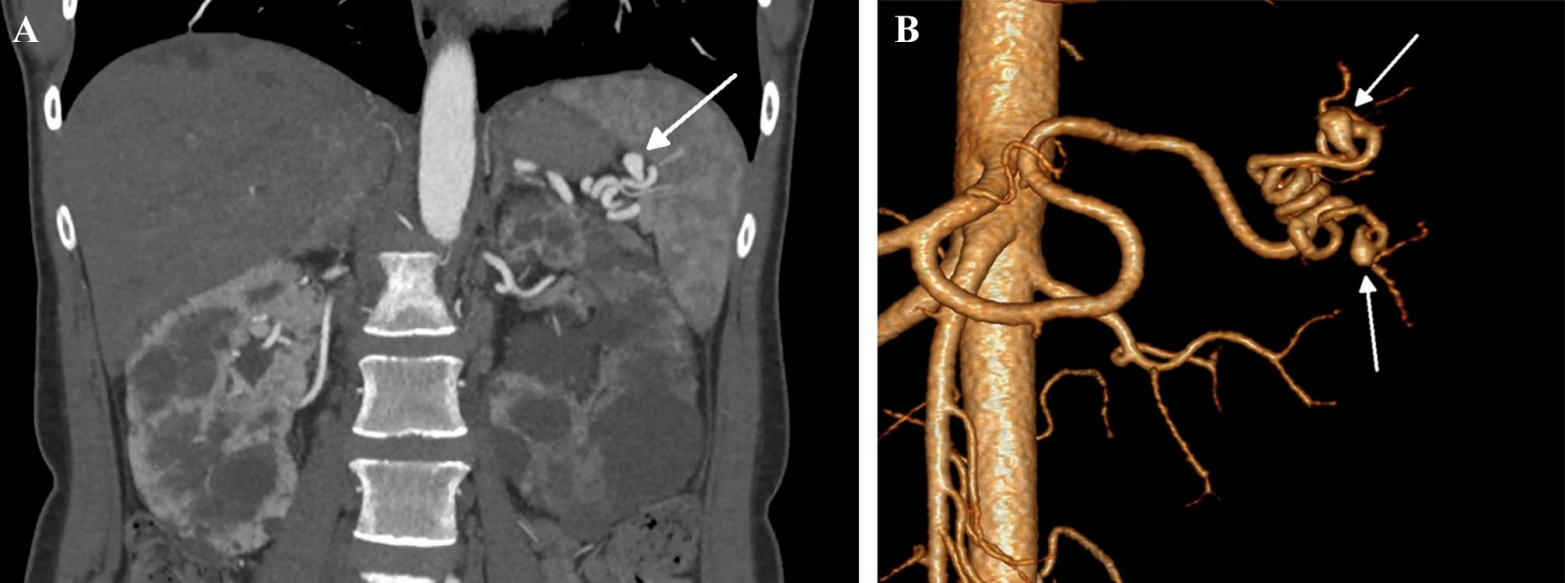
A Contrast-enhanced computed tomography of the abdominal cavity, coronal plane. Aneurysm of the splenic artery (arrow). Polycystic kidneys. B Contrast-enhanced computed tomography (3D reconstruction). Two aneurysms of the spenic artery (arrows)
